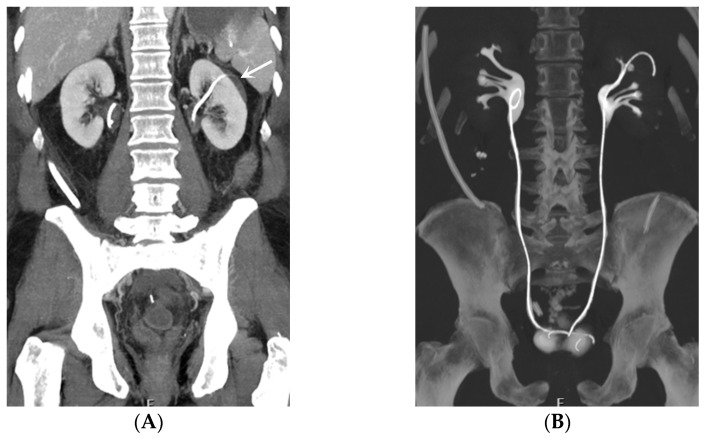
Bilateral stenting of the ureter. Coronal CT image (A) and coronal MIP image (B). On the left side, the device penetrates the renal parenchyma, causing the formation of a thin subcapsular collection (arrow).
